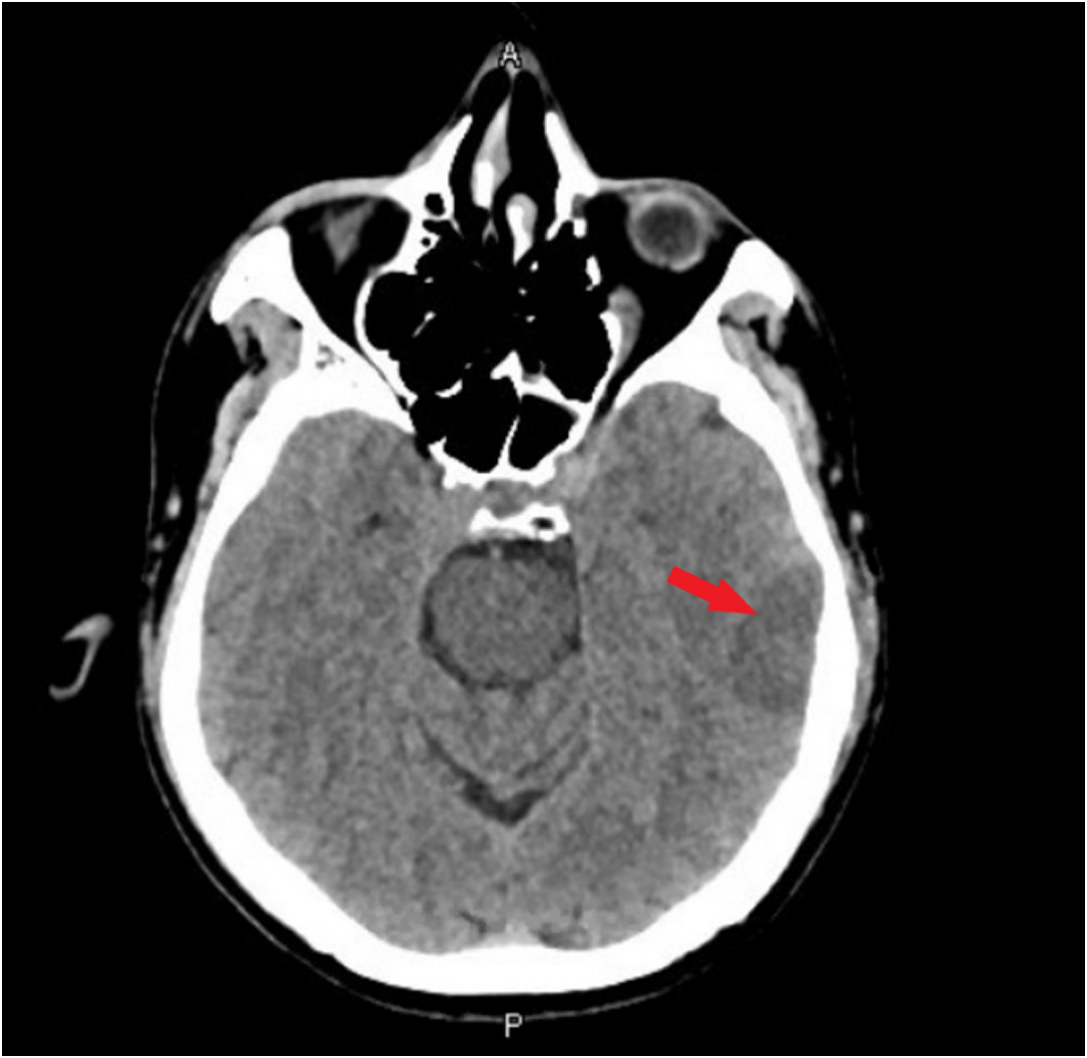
CT Head showing hypodense lesion in left temporal lobe (arrow) possible ischemic foci.
